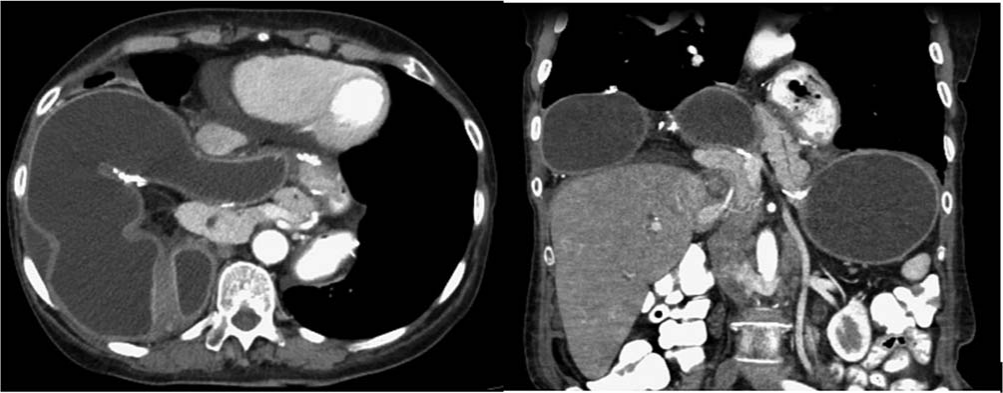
CT scan—the gastric pouch, gastrojejunal anastomosis, remnant stomach, and a portion of the pancreas were located within the thoracic cavity above the diaphragm.
